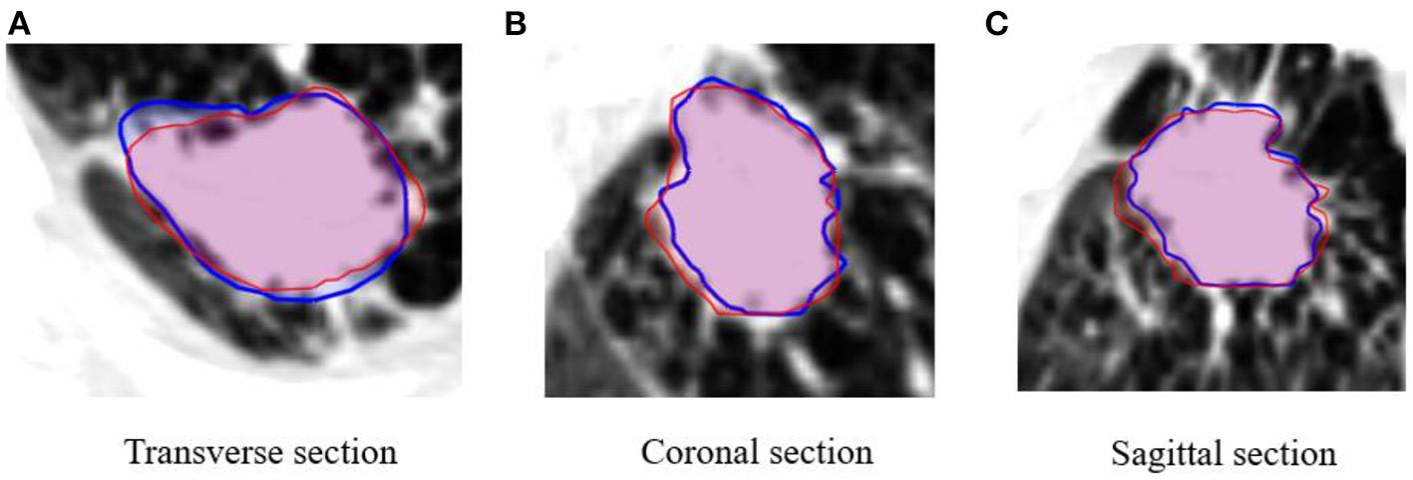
Comparison of contours between ITV on the 4-dimensional CT (ITV_4DCT_, red) and ITV prediction (ITV_predict_, blue) in different views of patient images.
